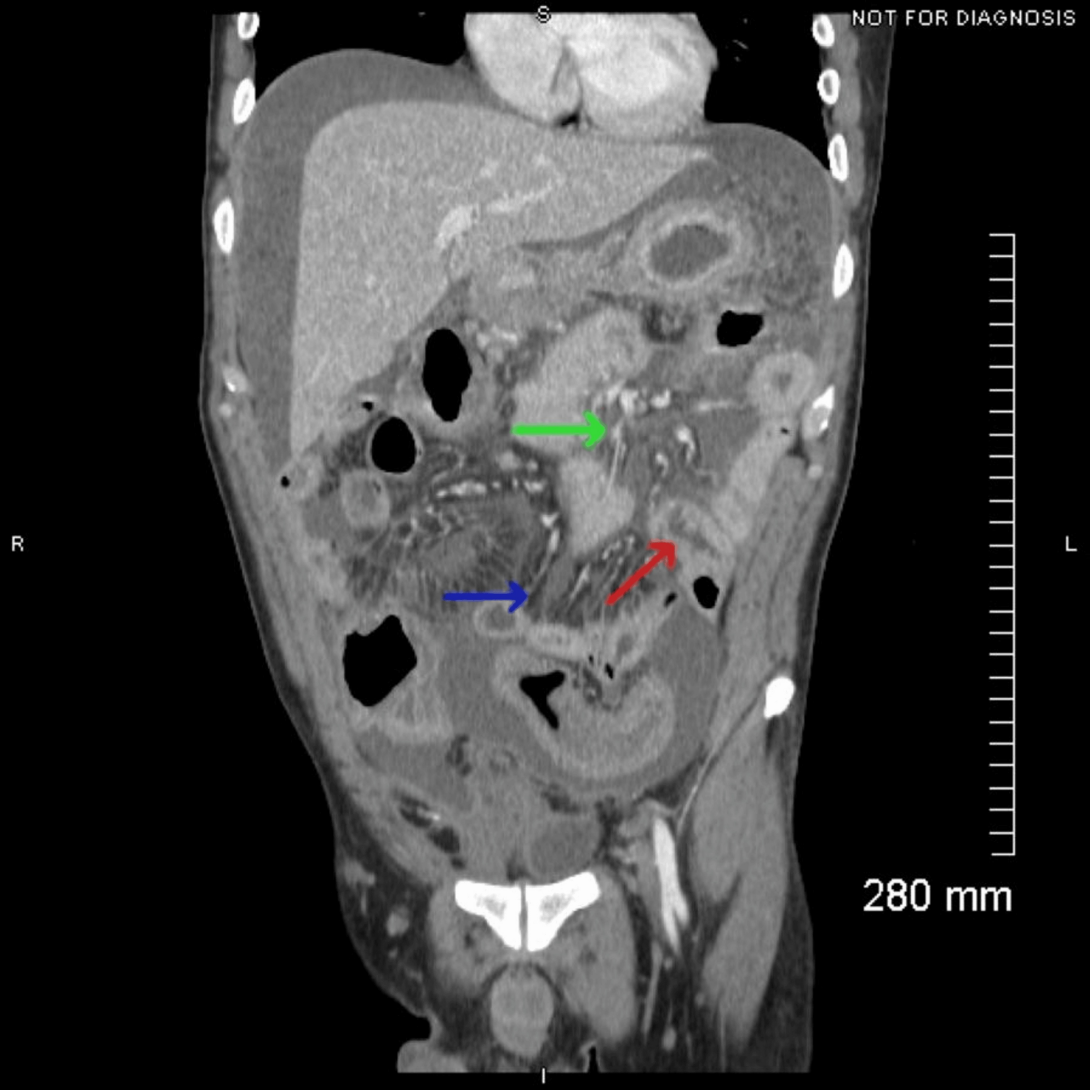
Bowel wall thickening with mesenteric nodularity and inflammatory change Coronal contrast-enhanced CT of the abdomen and pelvis demonstrating segmental bowel wall thickening (red arrow), mesenteric nodularity (green arrow), and surrounding inflammatory fat stranding (blue arrow). CT: computed tomography
